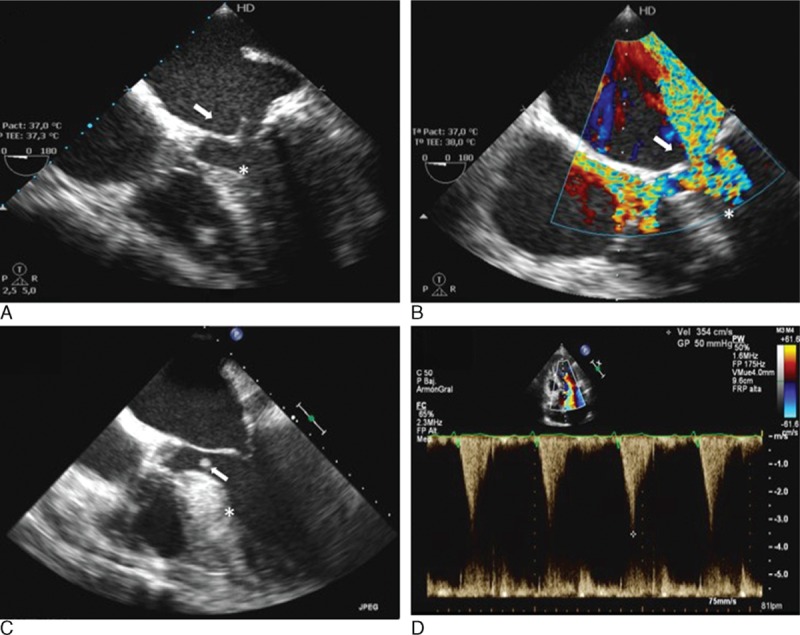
Examples of IE in 2 HCM patients. A and B, Mitral valve endocarditis. A, Transesophageal echocardiogram, 4 chamber view, 0°. An oscillating 16-mm vegetation is observed on the left atrial side of the anterior mitral leaflet (white arrow). Septal hypertrophy of 16 mm (white asterisk). B, Transesophageal echocardiogram, 5 chamber view, 0°. Color Doppler across the mitral valve with evidence of severe mitral regurgitation (white arrow), as well as flow acceleration noted in the left ventricle outflow tract (white asterisk). C and D, Infective endocarditis affecting the basal interventricular septum. C, Transesophageal echocardiogram, 4 chamber view, 0°. An 8- by 4-mm vegetation is evidenced 20 mm below the aortic valve (white arrow). Severe septal hypertrophy with a maximal wall thickness of 27 mm (white asterisk). D, Pulsed wave Doppler at the left ventricular outflow tract. Maximum gradient of 50 mm Hg and peak velocity of 3.5 m/s, with the characteristic dagger-shaped appearance seen in obstructive HCM. HCM = hypertrophic cardiomyopathy, IE = infective endocarditis.
